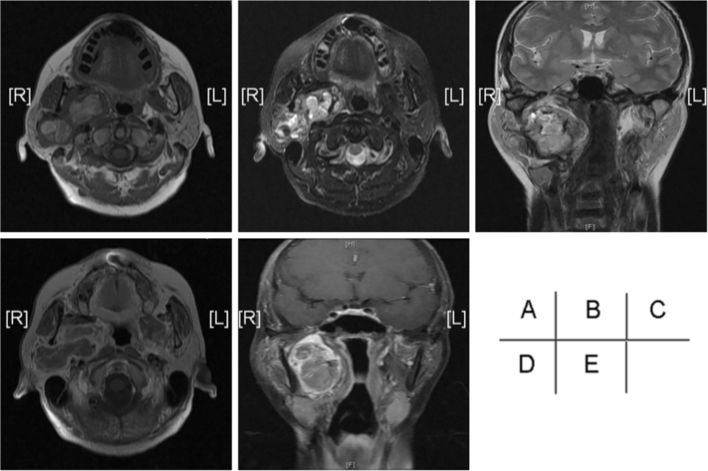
MR images. A, B, C is MRI plain scan. A is transverse TIWI, B is the axial pressure grease T2WI and C is coronal T2W1. Figure D and E is enhanced MRI, D is transverse T1W1 and E is coronal fat suppression T1W1.
